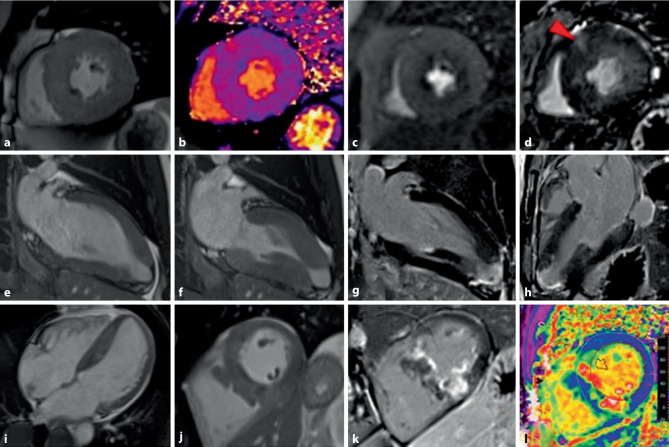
Features of hypertrophic cardiomyopathy in cardiac magnetic resonance imaging (CMRI). a-d patient 1, e-h patient 2, i-l patient 3. *Patient 1: a 52-year-old patient with a symmetrical form of hypertrophic cardiomyopathy (HCM).*
